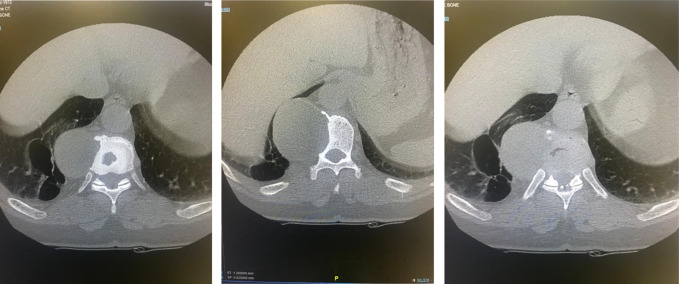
Radiograph showing the axial view of the bony changes caused by the pseudoaneurysm.
